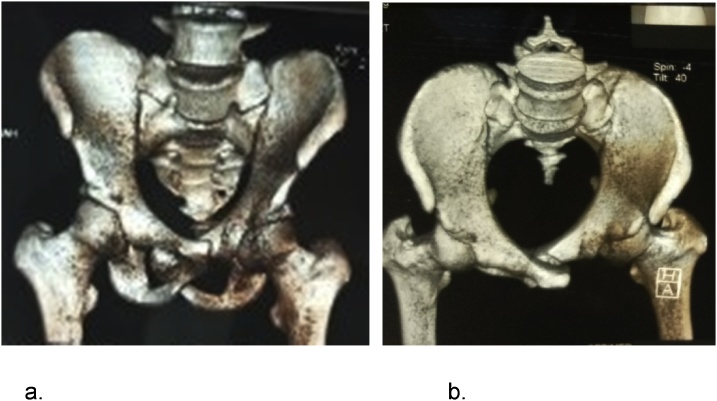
Pelvic CT scan showing overlapped pubic symphysis, with the left pubic symphysis locked into the right obturator foramen. a. Front view. b. Top view.
